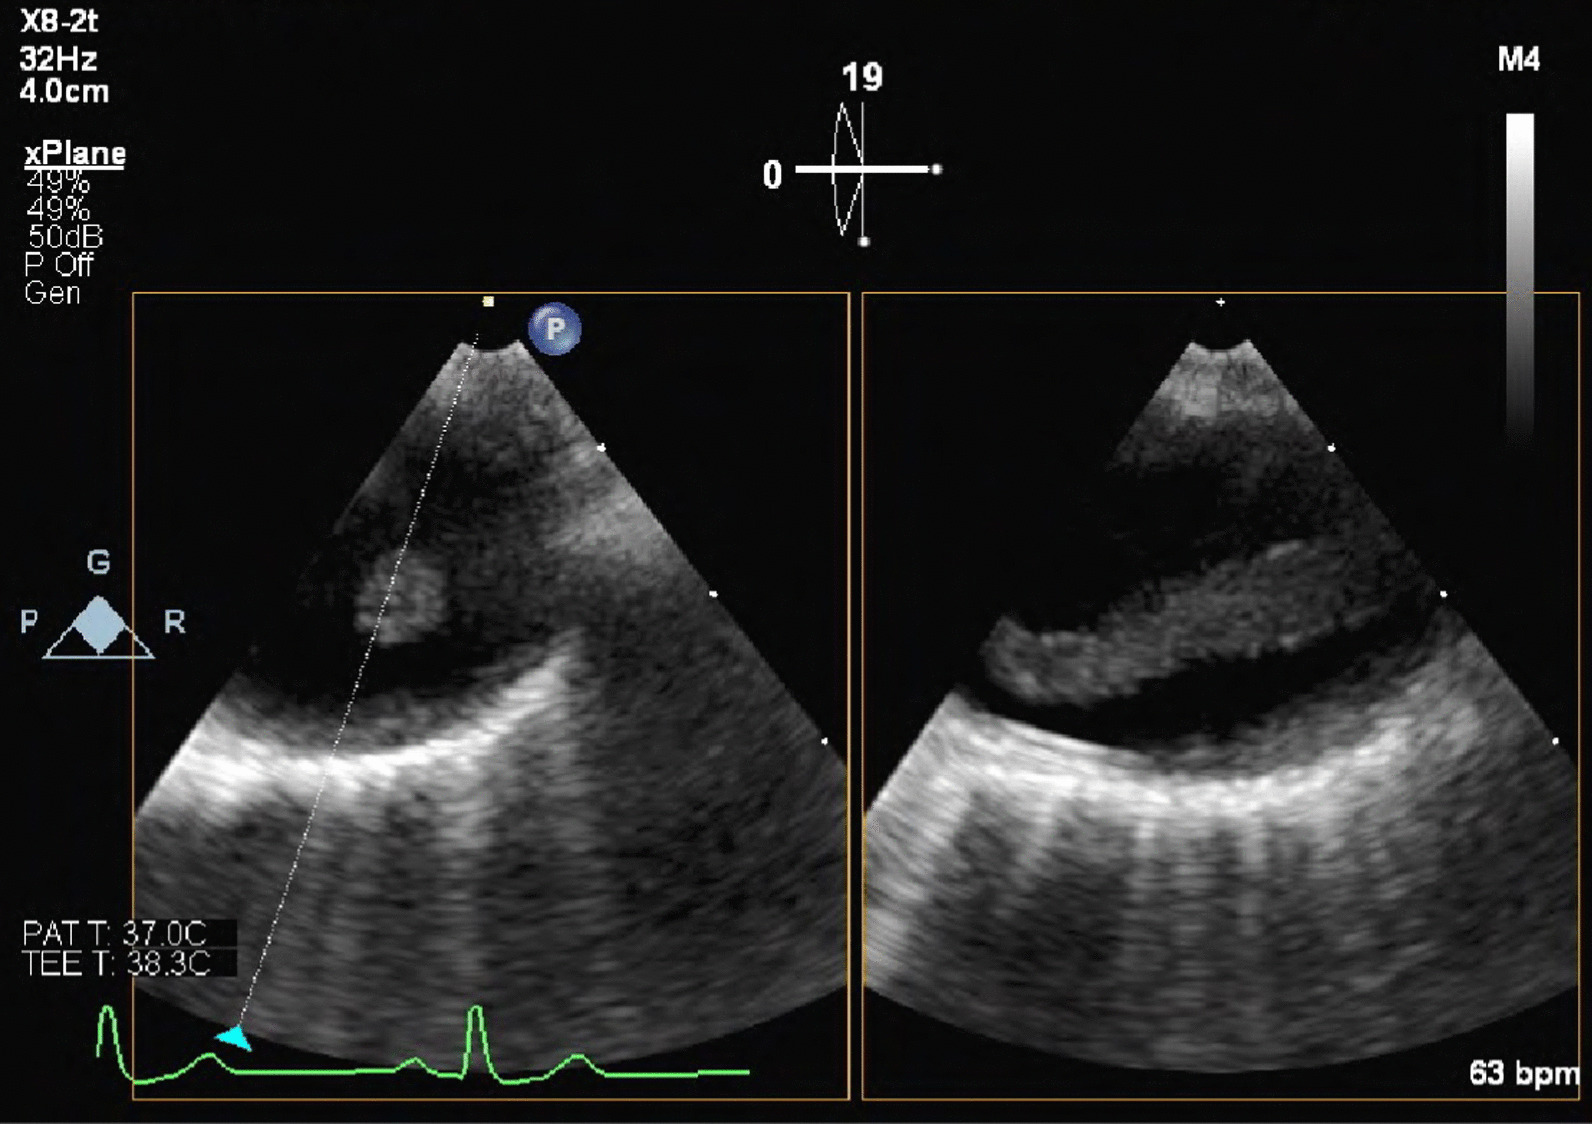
Transesophageal echocardiography showing a fluttering cord-like thrombus in the aortic arch extending to the left common carotid artery
